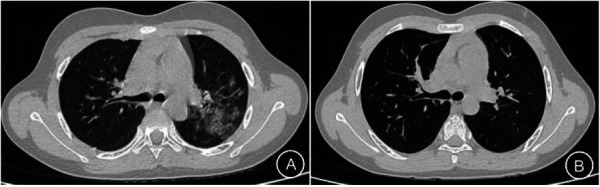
Chest CT imaging of a child with CPP (12-year-old boy, admitted with cough for 9 days and exacerbation accompanied by fever for 4 days). (A) Initial CT demonstrates multiple patchy opacities in the left upper lobe with indistinct margins. (B) Follow-up CT 13 days later shows significant reduction, fading, and near-complete resolution of the patchy opacities in the left upper lobe compared to the initial scan.
